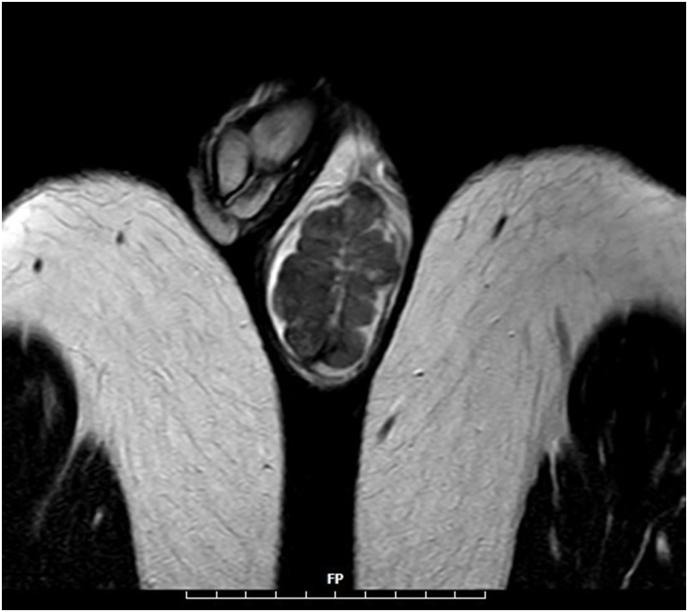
The MRI of testis: Isointense mass on T1-weighted images and hypointense mass on T2-weighted images, relative to the normal parenchyma.
